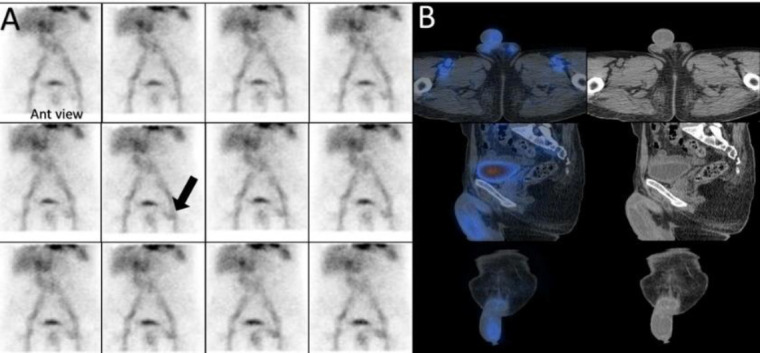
A zone of activity in the left inguinal region fluctuating over time (arrow-A) proven to be penile activity on SPECT/CT (B)
